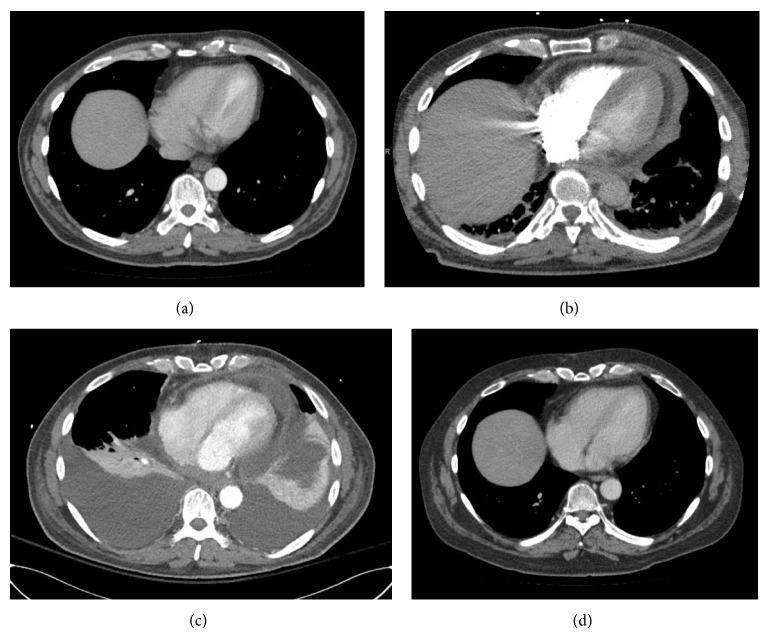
CT chest on admission showed pericardial thickening, moderate-sized pericardial effusion, and adjacent inflammatory changes within the epicardial fat and mediastinum (b), which were new compared to the prior study (a). New large bilateral pleural effusion with associated compressive atelectasis in the lower lungs and stable pericardial effusion is observed on day 10 (c), which resolved after systemic steroid treatment (d).
